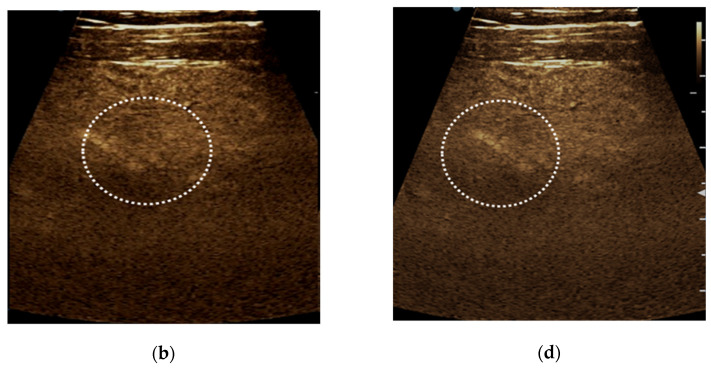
Example of LI-RADS-2 liver lesion. Regenerative nodule <10 mm depicted by linear probe exam in a patient with hepatitis C cirrhosis. The ultrasound exam shows a nodular liver surface and a subcapsular hyperechoic liver lesion (a). On contrast-enhanced ultrasound (CEUS) the nodule shows the isoenhancing aspect in the arterial (b), portal-venous (c) and late phase (d).
